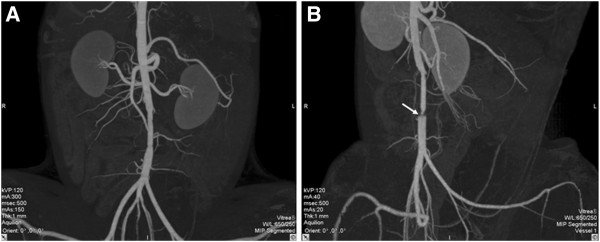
CT images taken 24 weeks after the surgery(i.e.,shortly before the sample harvest.) (A) A PU graft showing excellent patency, smooth internal wall, and absence of stenosis. (B) A PTFE graft showing patency, yet clear stenosis at the distal anastomotic site (marked with white arrow).
